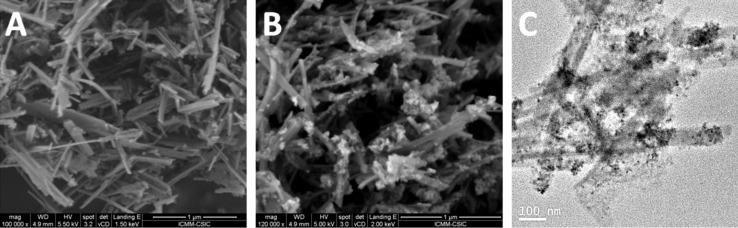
FE-SEM images of SiO_2_-SEP-CTA (A) and ZnO/SiO_2_-SEP (B) heterostructures, and TEM image of ZnO/SiO_2_-SEP heterostructure (C).
